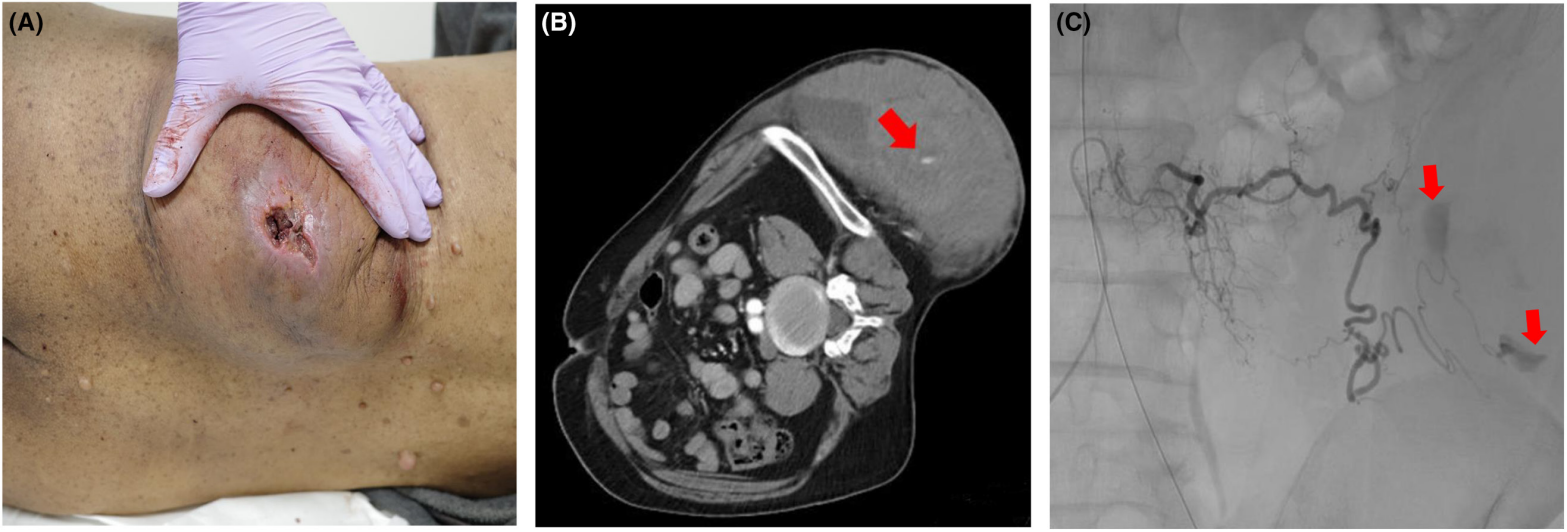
(A) Giant mass in the left lateral abdomen. (B) Contrast‐enhanced computed tomography revealed vascular leakage within the tumor (red arrow). (C) Emergency interventional radiology also showed vascular leakage (red arrows).
